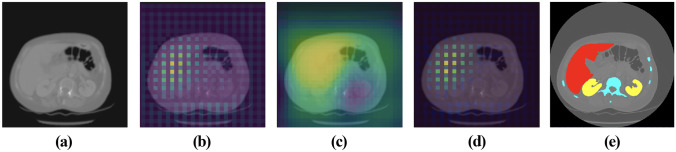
A representative visual effect of the LK attention module. a The CT scan input. b The upsampled feature map at the middle scale of the decoder. c The attention map. d The feature map after multiplying with the attention map. e The GT labels
